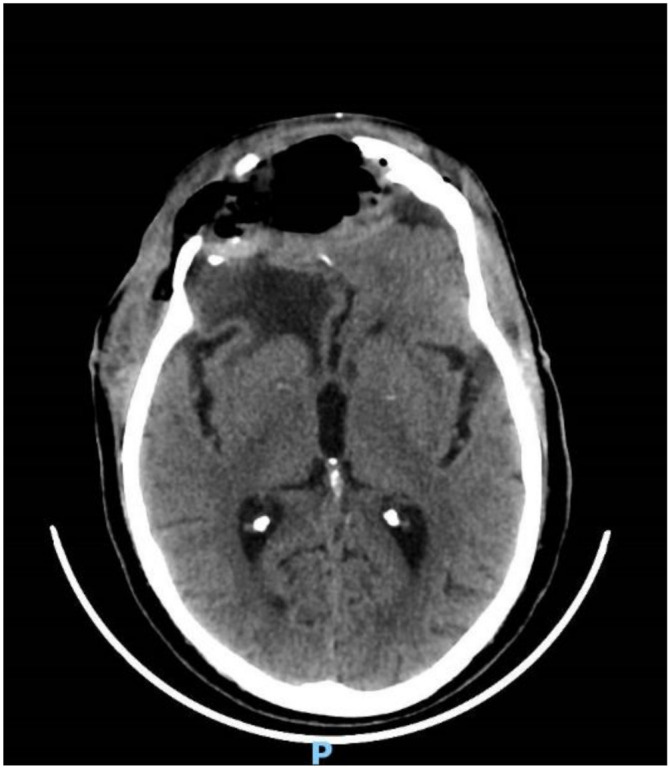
CT head scan showing evacuation of the empyema and post-operative pneumocephalus.
